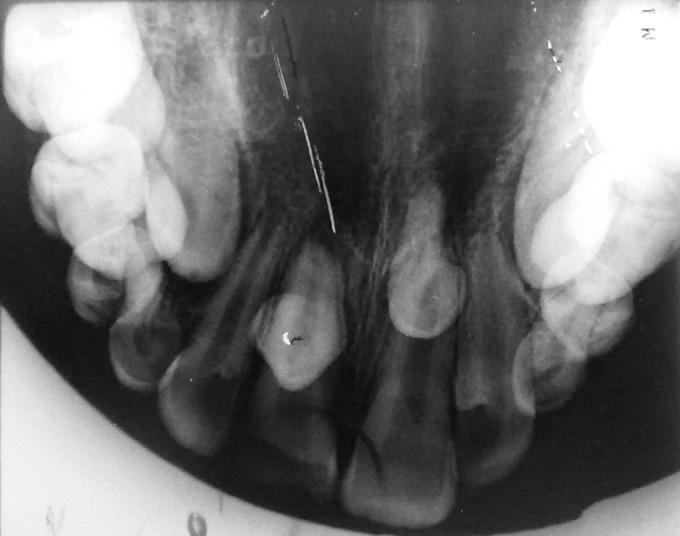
Maxillary occlusal radiograph revealing two supernumerary teeth (case I)
